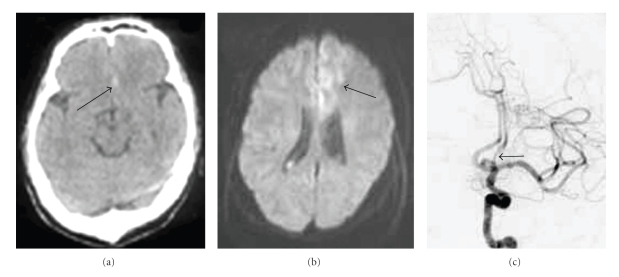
Imaging findings on the day of onset. (a) Computed tomography shows very thin subarachnoid hemorrhage on the left side of the interhemispheric fissure (arrow). (b) Magnetic resonance imaging (diffusion image) reveals infarction of the left medial frontal region (arrow). (c) Left internal cerebral artery angiography reveals stenosis (string sign) of the A_2_ segment (arrow).
